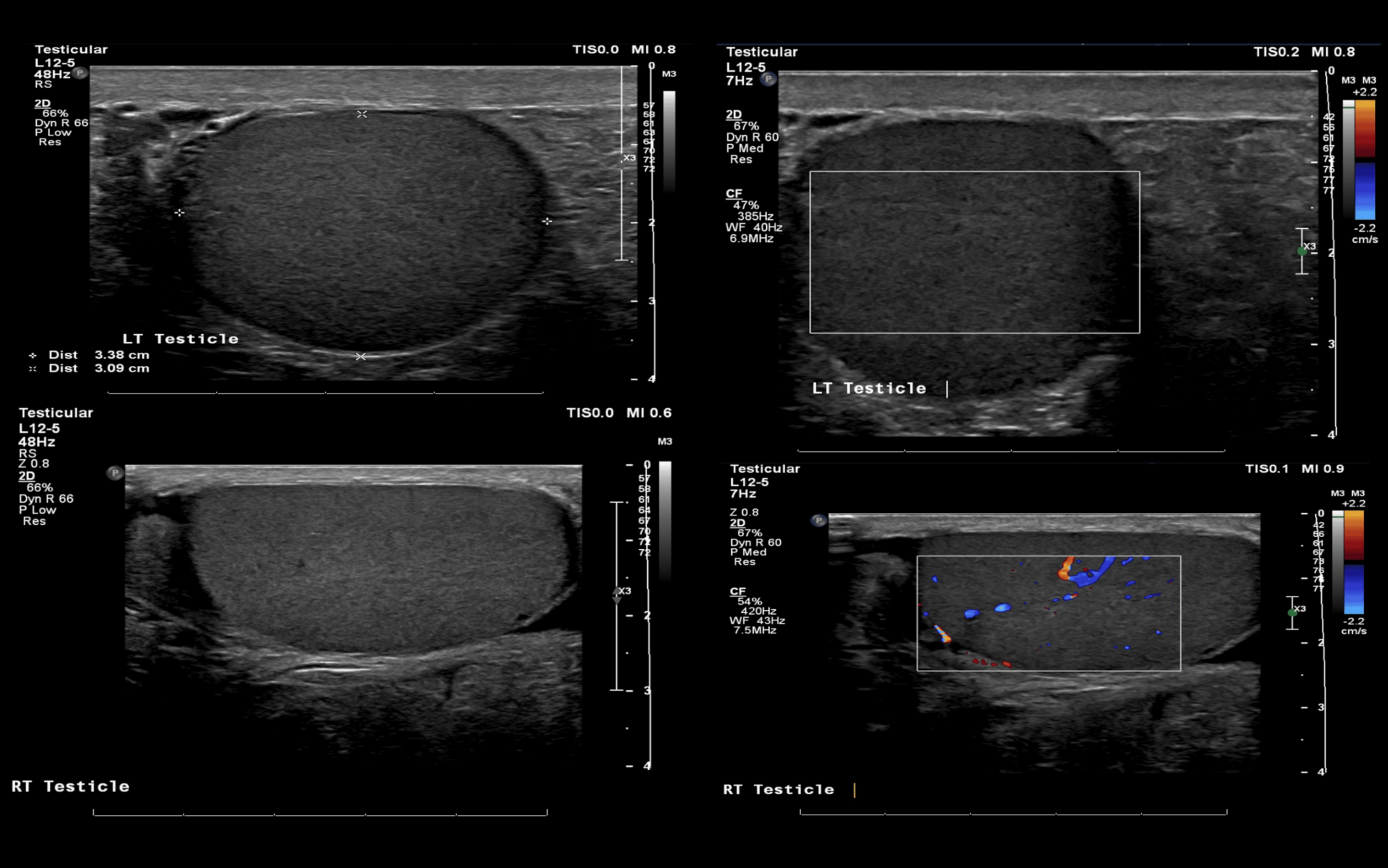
Scrotal ultrasound showing no vascularity in the left testis.
